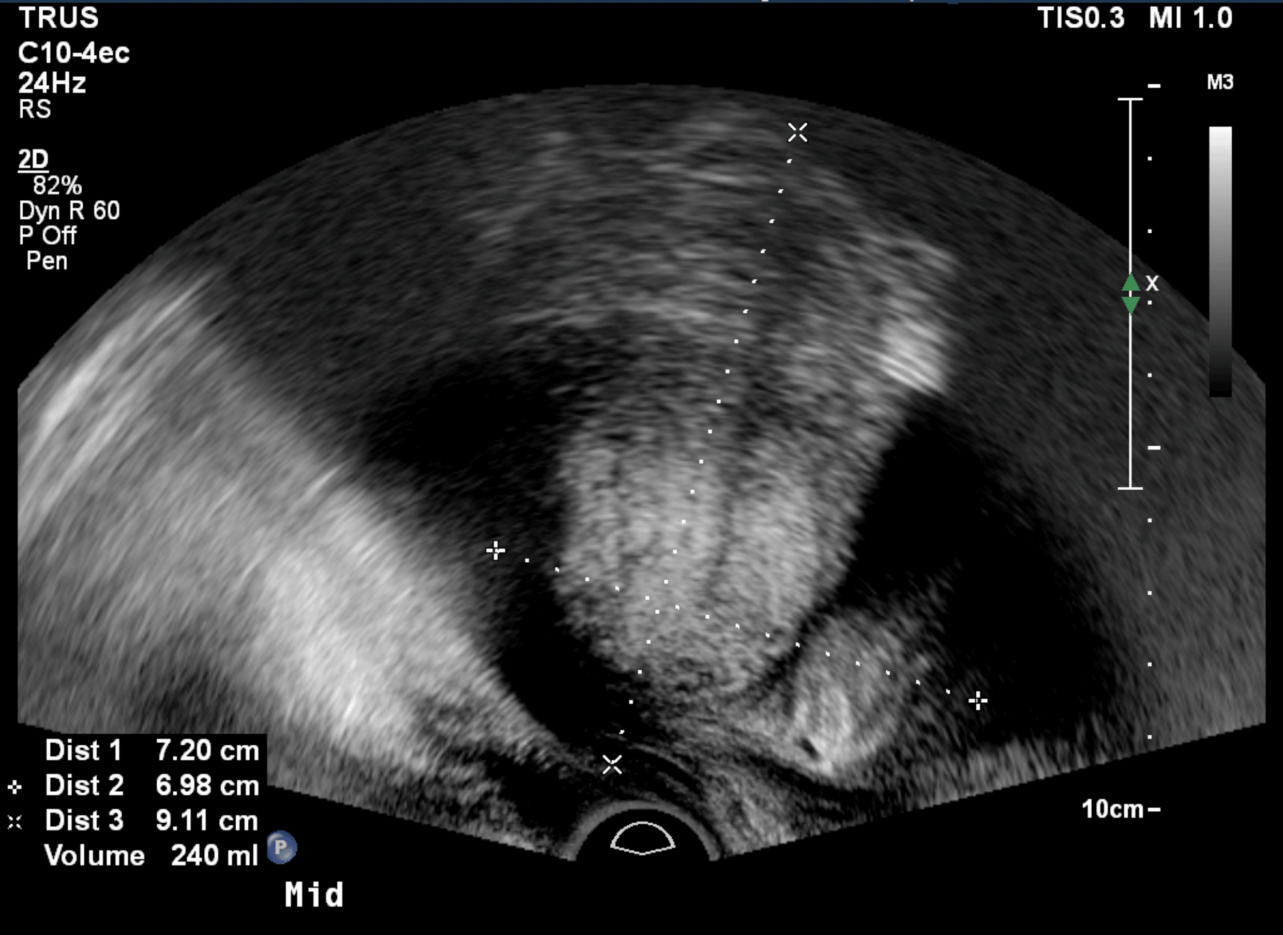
Case of failed fusion Large prostate size exceeded the sonographic field of view with poor penetration, limiting visualization of appropriate landmarks for fusion.
